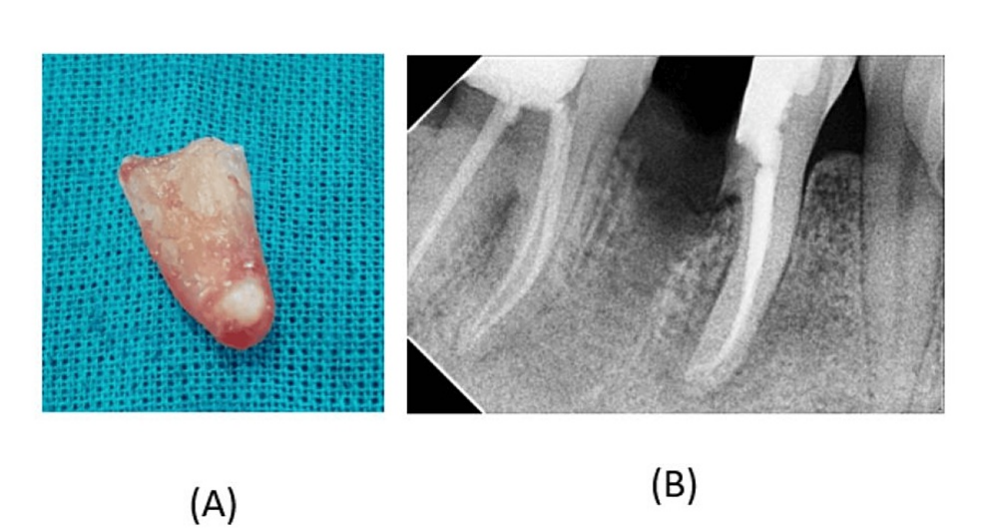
(A) Hemisection of the distal root of tooth number 46; (B) postoperative radiograph of tooth 46
